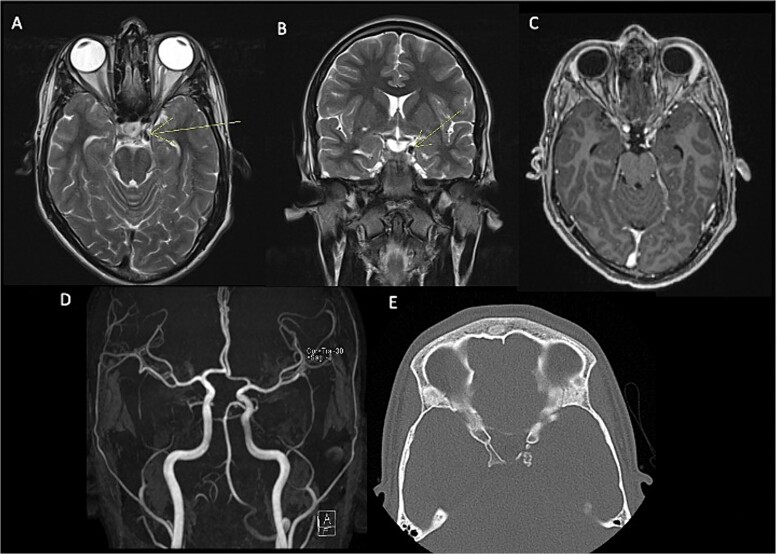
Pre-surgical MRI (A–C) showing the presence of a lesion with low signal intensity on T2-weighted sequences (A, B) of 0.7 × 0.6 cm adjacent to anterior clinoid process and tents, concerning the oculomotor left cistern, with peripheral enhancement in post-contrast sequences (C) contacting the left third cranial nerve. No evidence of aneurysms in angiogram MRI (D). Head CT scan (E) with a calcified exophytic lesion concerning the left anterior clinoid.
